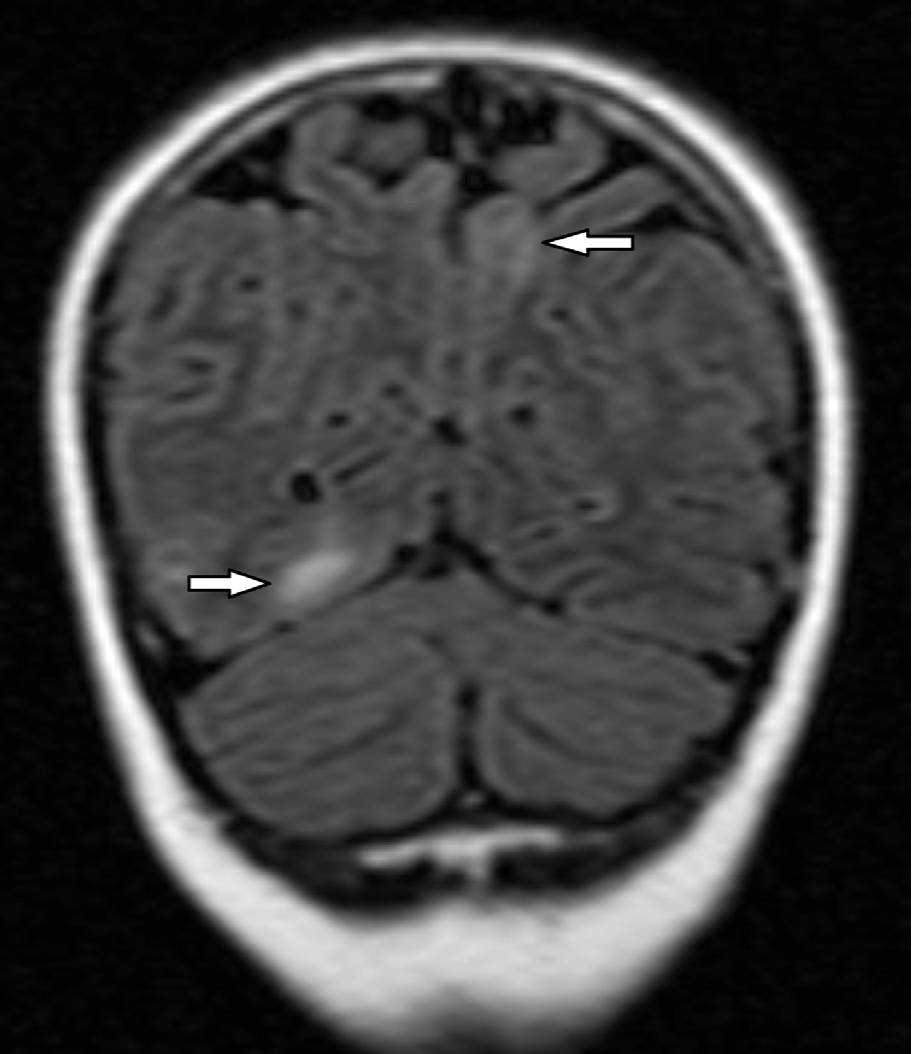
Coronal fluid attenuation inversion recovery (FLAIR) MRI through the posterior brain showing bilateral patchy areas of high signal within the subcortical white matter of right occipital lobe and left parietal lobe.
